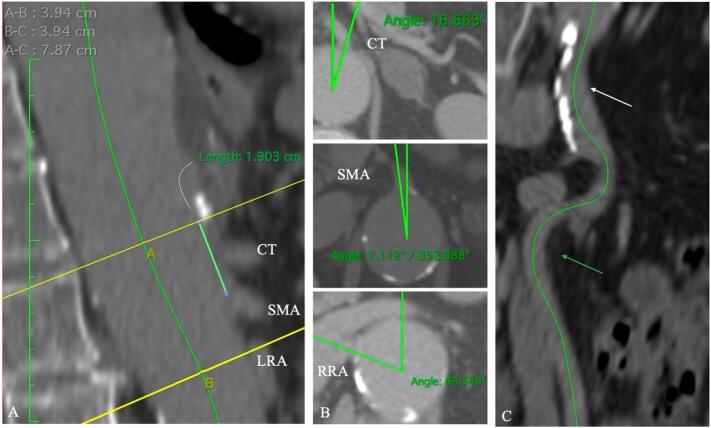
Anatomical features of the preoperative computed tomography angiography (CTA). A) The A-line passed through the ostium of the celiac trunk (CT), whereas the B-line passed through the ostium of the left renal artery (LRA). The distance between the CT and the superior mesenteric artery (SMA) was 19.3 mm, while the distance between the CT and the LRA was 39.4 mm; B) Angulation of the ostia of the visceral vessels relative to the major longitudinal axis passing through the aorta was measured as follows: CT: 16.6°, SMA: 352.8°, LRA: 82°, right renal artery (RRA): 292°; C) Right iliac axes features, with minimal calcifications at the level of the common iliac artery (white arrow) measuring 2.1 mm in thickness and an absence of calcification at the level of the external iliac artery (green arrow). (For interpretation of the references to colour in this figure legend, the reader is referred to the web version of this article.)
